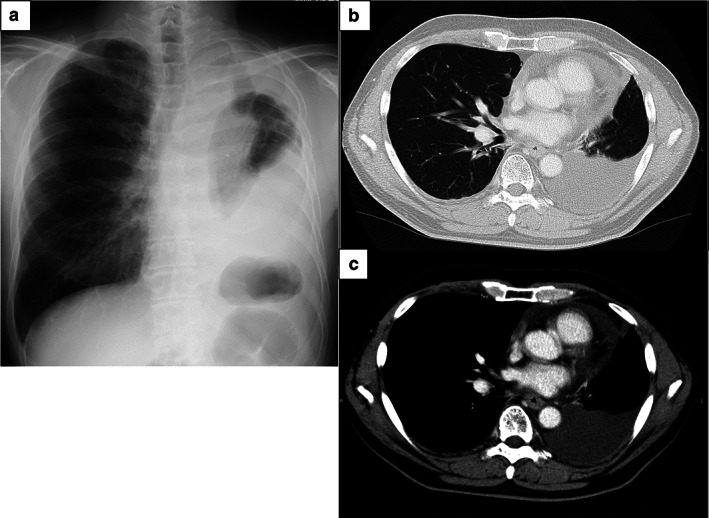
(a) Chest X‐ray showed decreased permeability in the lower left lung field and unaffected trachea; and (b–c) chest computed tomography (CT) scan showed a massive left pleural effusion and pericardial effusion.
